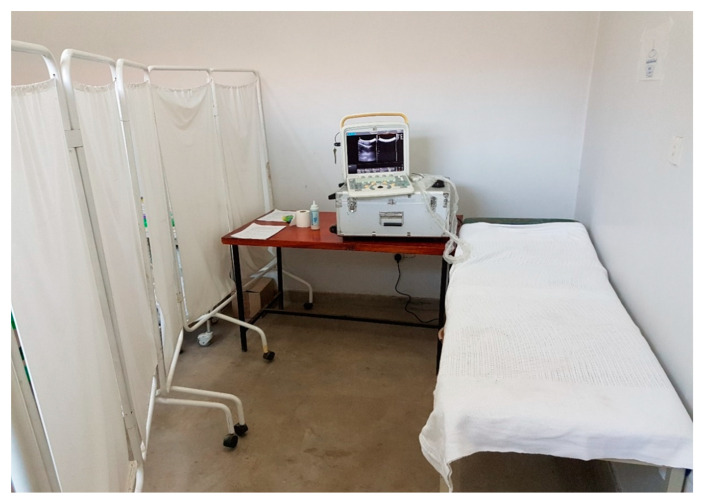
The portable Chison Q5 ultrasound scanner in an examination room.
